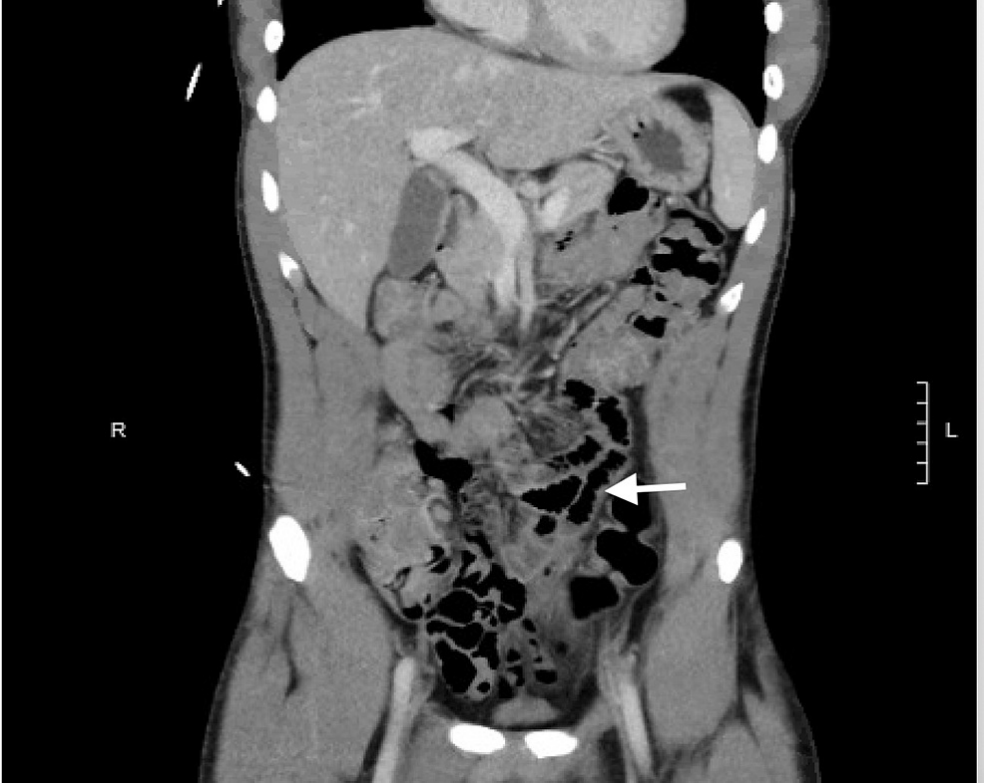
CT scan showing reactive small mesenteric lymphadenopathy and circumferential wall thickening of the colon with liquid stool noted in the rectum minimal surrounding fat stranding suggestive of pancolitis.
